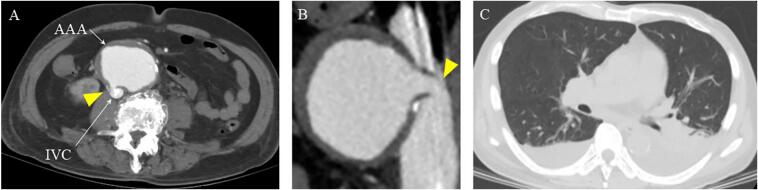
Preoperative Computed Tomography. (A, B) Large aortocaval fistula was observed and the inferior vena cava was contrasted in the arterial phase (shown as yellow arrows). (C) Bilateral chest pleural effusion. Abbreviations: AAA, abdominal aortic aneurysm; IVC, inferior vena cava. (For interpretation of the references to colour in this figure legend, the reader is referred to the web version of this article.)
